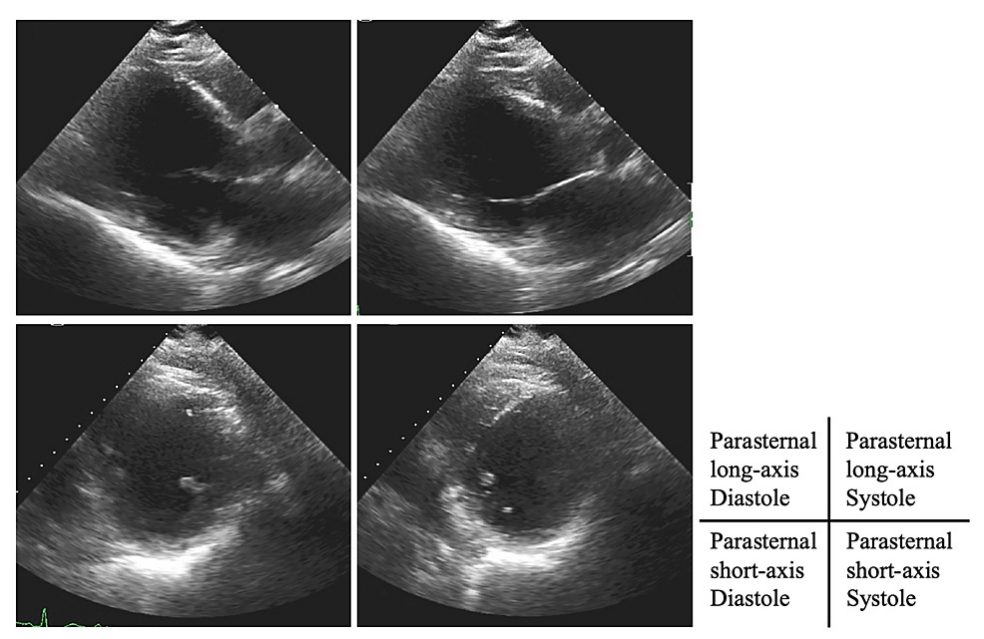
Echocardiography on admission Echocardiography showed a reduced ejection fraction of 19% and left ventricular dilation.
